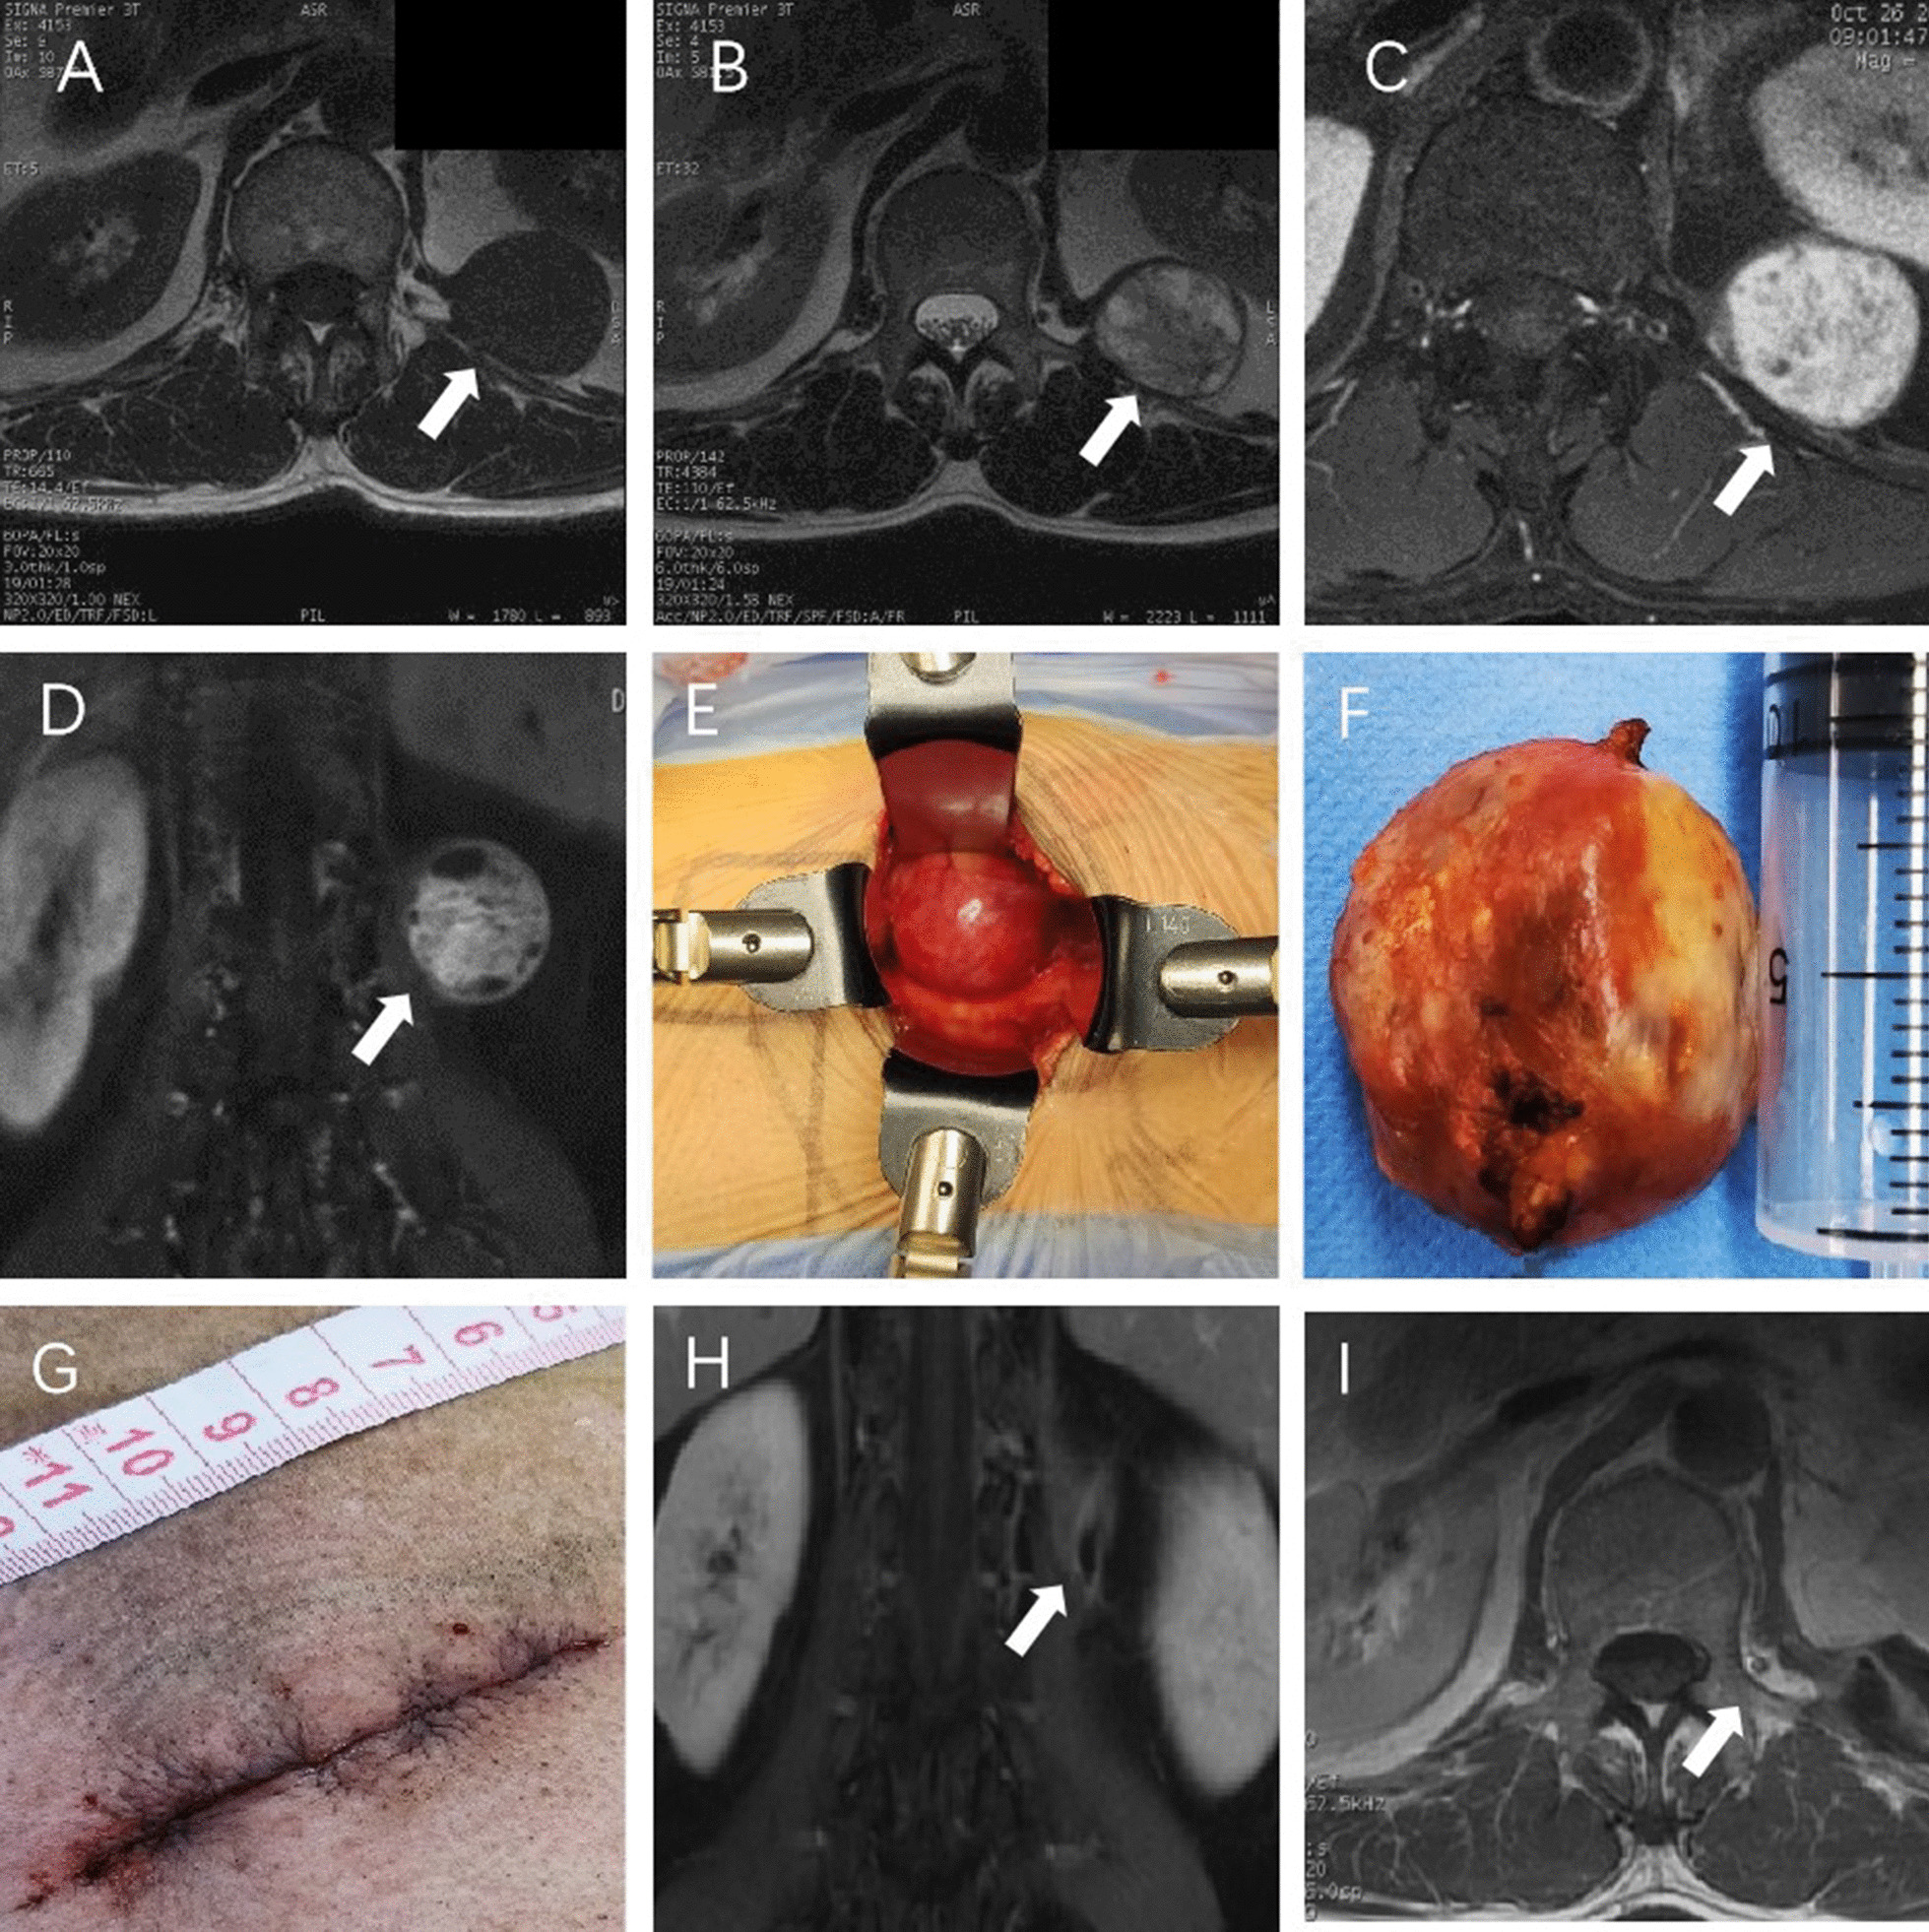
Microsurgical treatment of lumbar paravertebral tumors via lateral retroperitoneal approach. A MRI T1-weighted: a round-like paravertebral tumor (white arrow)at the posterior side of the kidney in the retroperitoneum, low signal. B MRI T2-weighted: small amount of mixed and uneven high signal, round-like tumor (white arrow). C MRI Gad-enhanced T1-weighted: axial view, a round-like tumor (white arrow) posterior to the kidney can be observed, significantly enhanced. D MRI Gad-enhanced T1-weighted: coronal view, a round-like tumor (white arrow) can be observed at L1–2 vertebral body level. E Intraoperative image: a 14 cm deep retractor fully exposes the tumor after retraction. F Postoperative image: The tumor was completely resected along the inner surface of the capsule, about 4 cm in diameter. G Postoperative image: the surgical incision length is about 7 cm. H, I Postoperative MRI image: image of retroperitoneal space, complete removal of the tumor (white arrow) located posterior to the kidney
